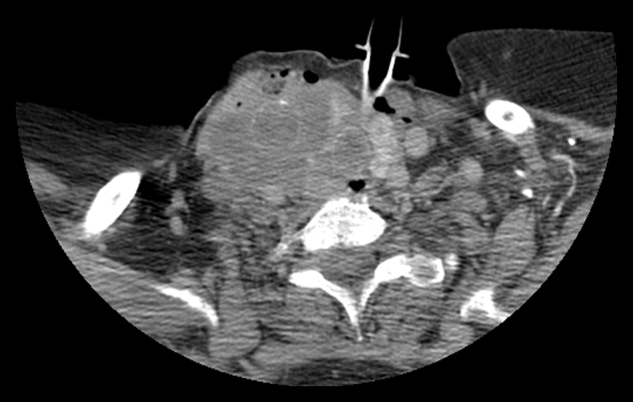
CT scan of the second patient, showing an infiltrative mass in the right thyroid lobe. Tracheotomy tube is also seen in the section.
